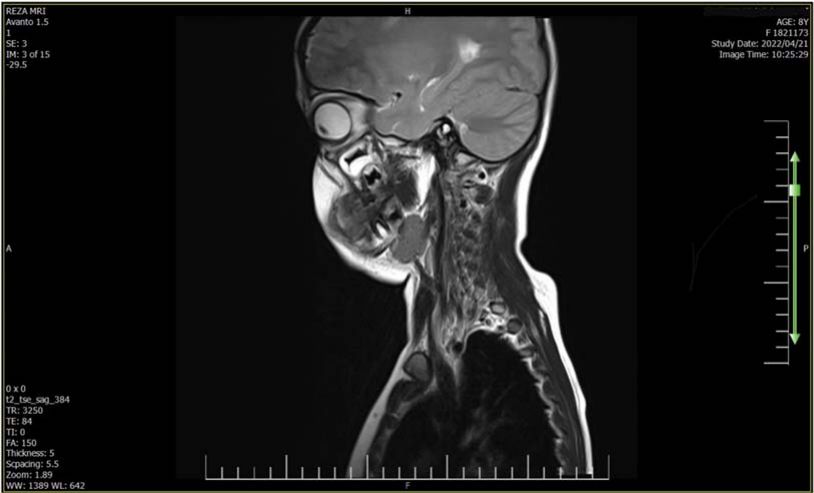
Present the MRI images which reveal a multiloculated nodular lesion in the intraluminal part of the trachea and also partly in the infra-glottic area, measuring 12×8 mm and taking up half of the tracheal lumen.
